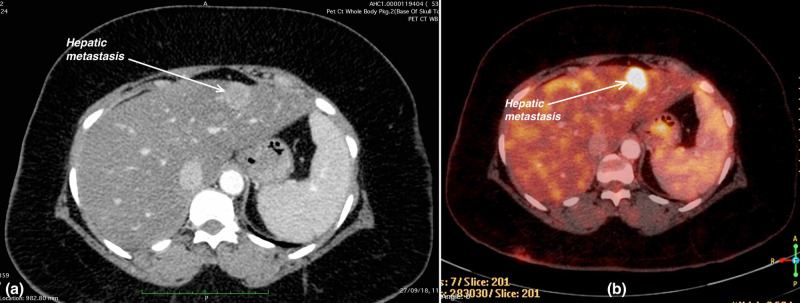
(a) Contrast-enhanced CT abdomen image shows a new solitary hepatic metastasis in the left lobe of the liver. (b) Corresponding fused PET-CT image shows a new metabolically active hypodense lesion of size 20 x 16 mm in the left lobe of the liver. PET-CT, positron emission tomography-computed tomography.
